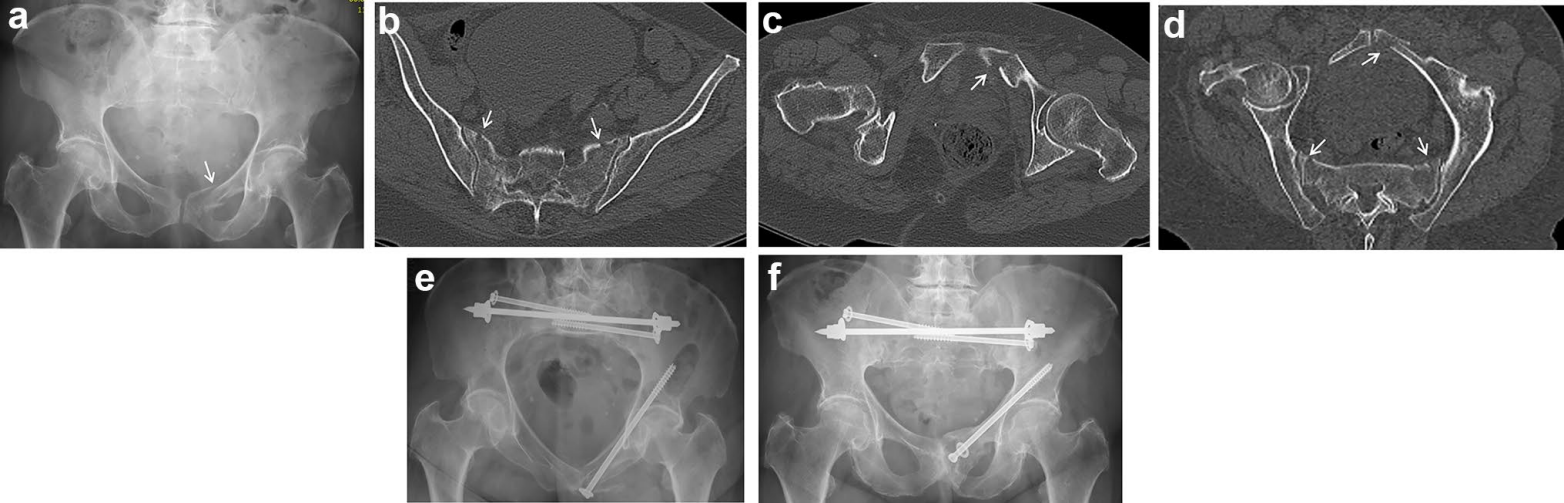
a–f A 77-year-old woman suffered severe pelvic pain after a fall at home. The a.p. pelvic overview shows a left superior and inferior pubic ramus fracture (arrow) (a). A transverse CT-cut through the sacrum shows bilateral non-displaced sacral alar fractures, complete on the right and incomplete on the left side (arrows) (b). Transverse CT-cut through the pubic symphysis confirms the left-sided superior pubic ramus fracture (arrow) (c). Oblique CT-cut though the level of the pelvic brim shows the posterior and anterior instabilities of the pelvic ring (arrows) (d). These fractures corresponded with a FFP type IIc. The patient was treated conservatively during 1 week. Due to continuing immobilizing pain, surgical stabilization was performed after 8 days. The fractures were fixed operatively with a transsacral bar and bilateral iliosacral screws. The pubic ramus fracture was stabilized with a retrograde transpubic screw. Postoperative pelvic inlet view (e). The a.p. pelvic overview more than one year after surgery shows complete healing of all fractures. There is a slight loosening of the retrograde transpubic screw (f)
